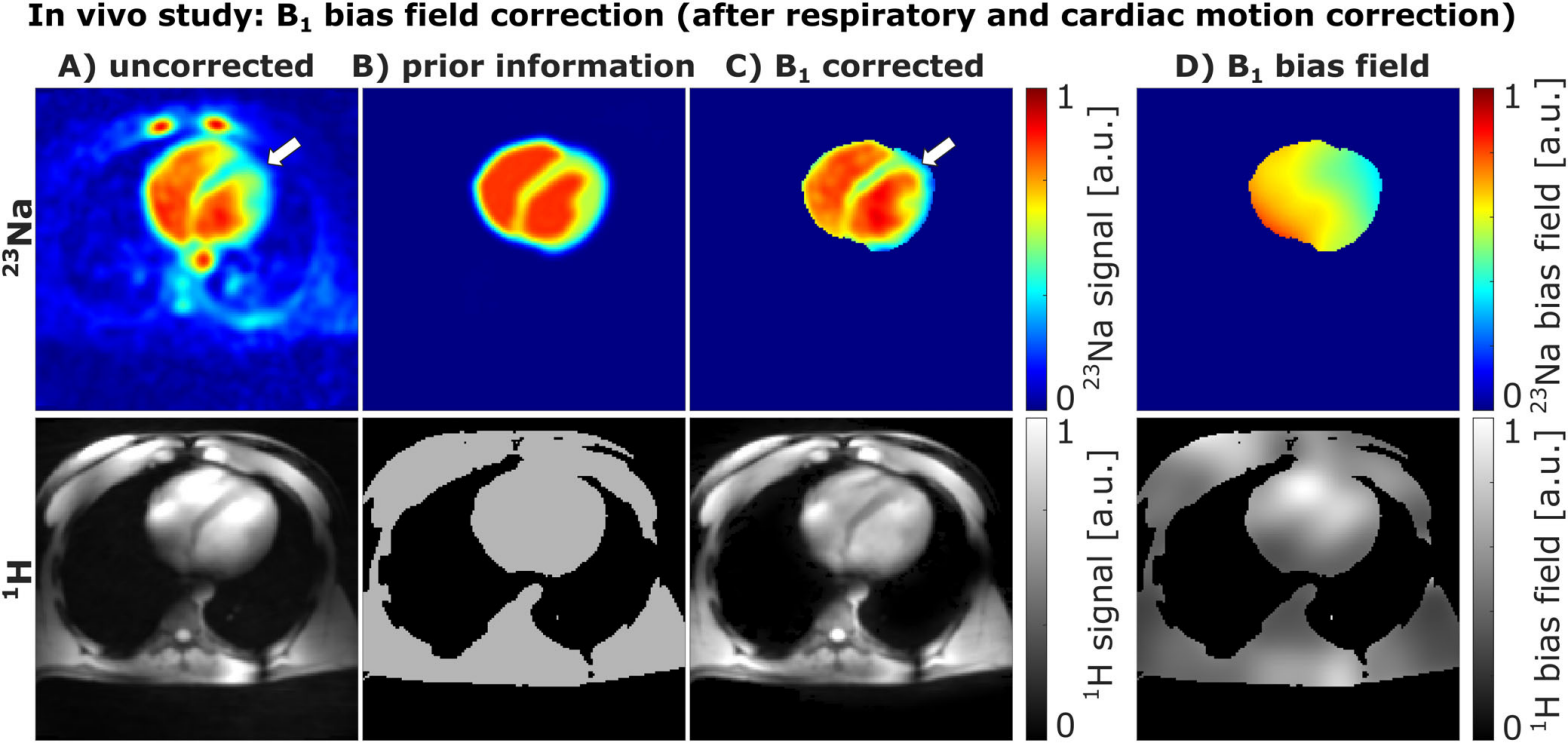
Influence of B_1_ bias field correction on ^23^Na and ^1^H images for one subject. (A) shows the respiratory and cardiac motion‐corrected ^23^Na and ^1^H image before B_1_ bias correction. For ^1^H MRI, a simple homogeneous support region (B) was used as anatomical prior to extract the ^1^H B_1_ bias field (D). After correction (C), the ^1^H image exhibits a more homogeneous signal distribution within the heart. For ^23^Na MRI, the prior information was generated based on ^1^H segmentations of the myocardium and blood (B). The estimated ^23^Na B_1_ bias field contained no anatomical structures (D). The B_1_ correction compensates for the signal loss on the top left of the heart (white arrow), leading to a more homogeneous signal distribution over the heart (C). Note that since the ^23^Na B_1_ bias field correction was applied only within the heart, the B_1_ corrected ^23^Na image (C) and the ^23^Na bias field (D) were masked accordingly.
